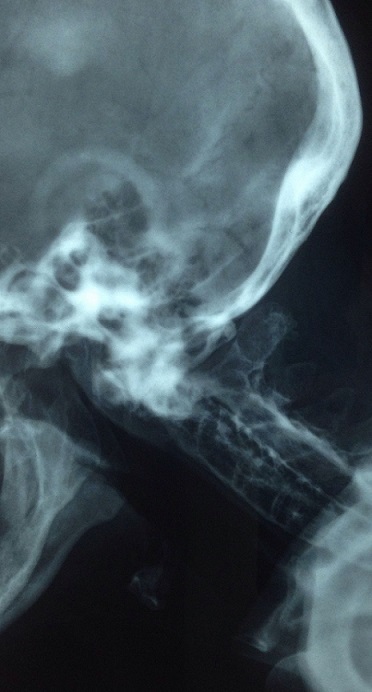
Radiograph of a cervical spine showing a vertical atlantoaxial subluxation complicating spondyloarthritis
